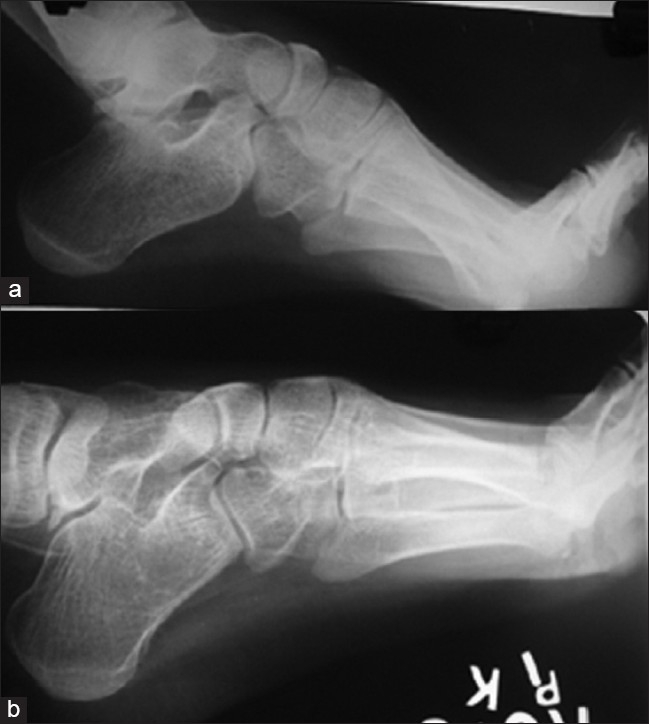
Lateral X-ray of foot (a) shows pre-operative deformity and post-operative X-ray of the same patient after Japas osteotomy
